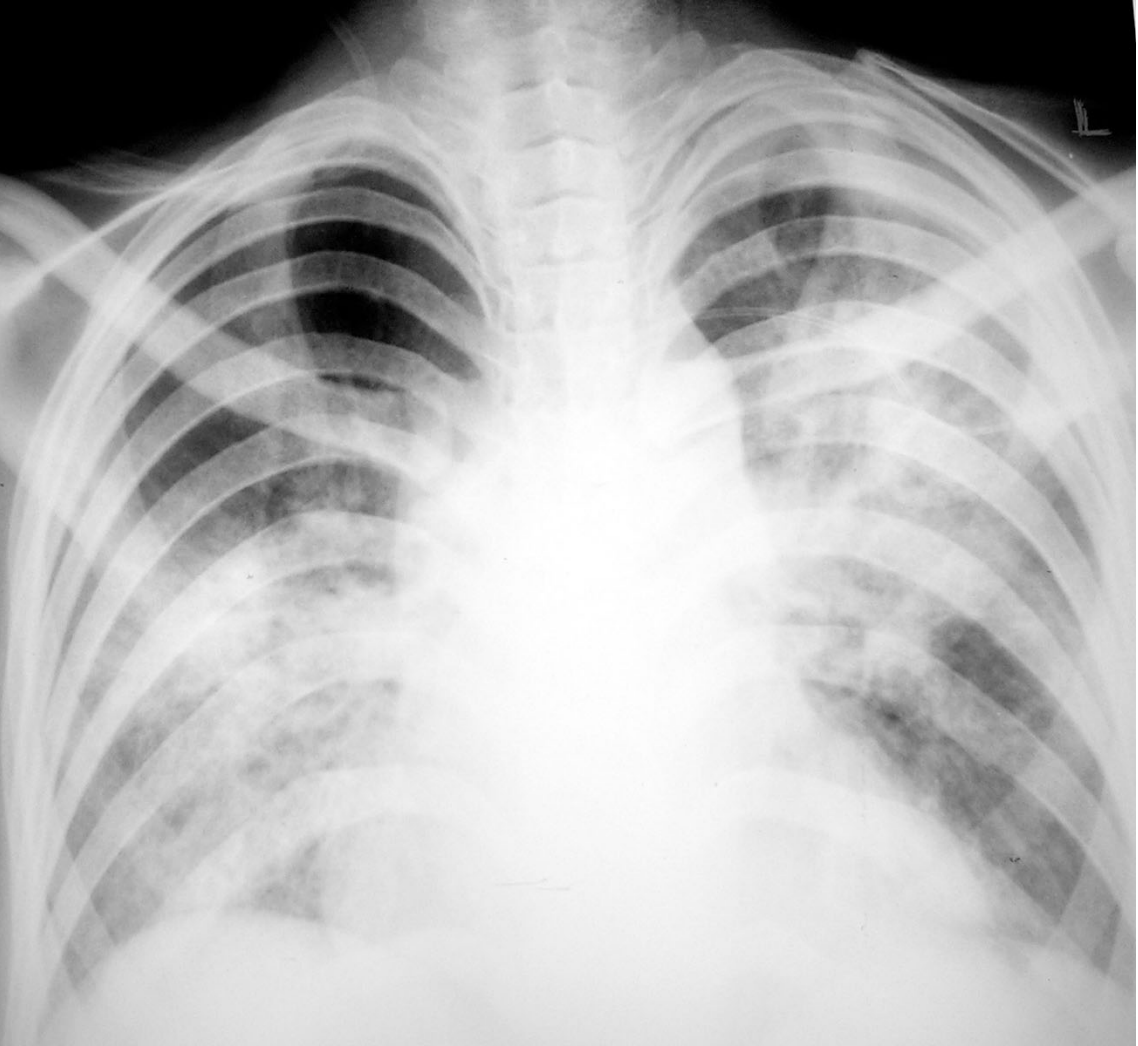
Chest radiograph shows bilateral perihilar alveolar opacities.
